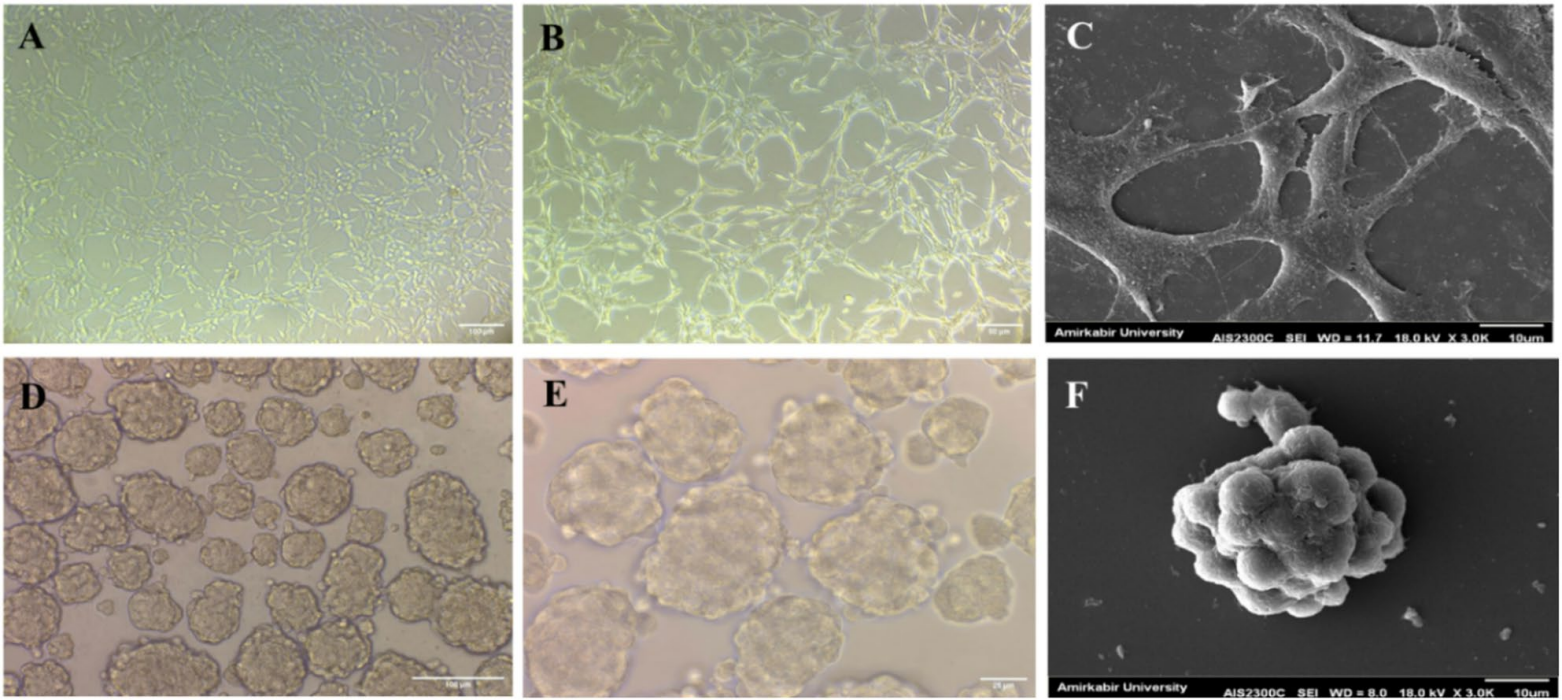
Morphology of CT-26 parental and spheroid cells. A, B, CT-26 parental cells cultured under 2D conditions; magnifications ×100 and ×200. C, SEM image showing the morphological features of CT-26 parental cells (scale bar = 10 μm). D, E, CT-26 spheroid cells derived from CT-26 parental cells and cultured in spheroid culture medium; magnifications ×200 and ×400. F, SEM image showing the structural characteristics of CT-26 spheroid cells (scale bar = 10 μm)
